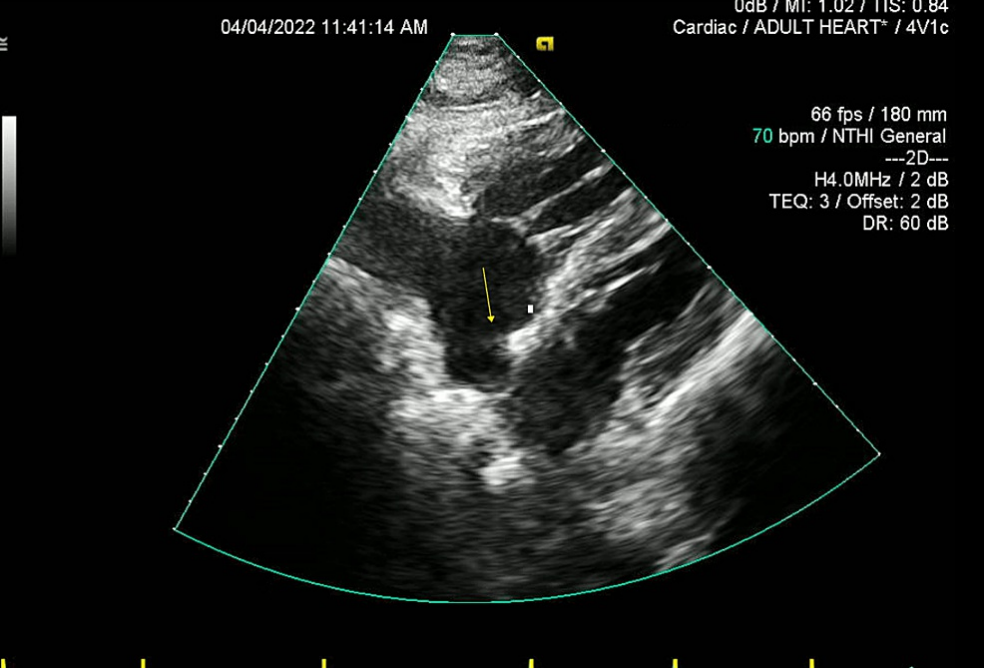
Transthoracic ECHO delineating the aneurysmal inter-atrial septum
